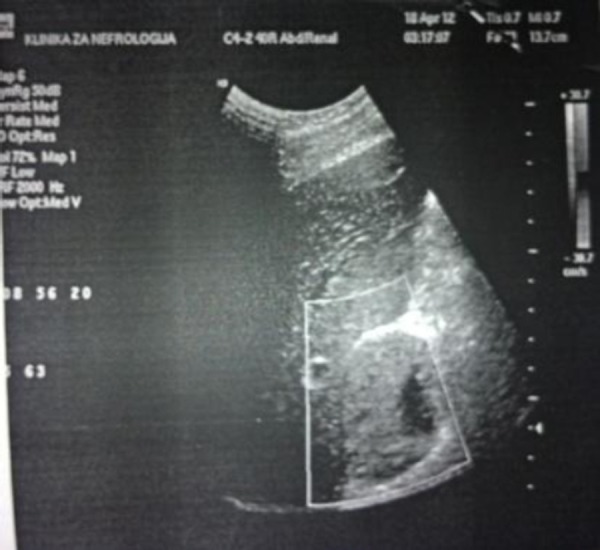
Right suprarenal mass on abdominal ultrasound*.
